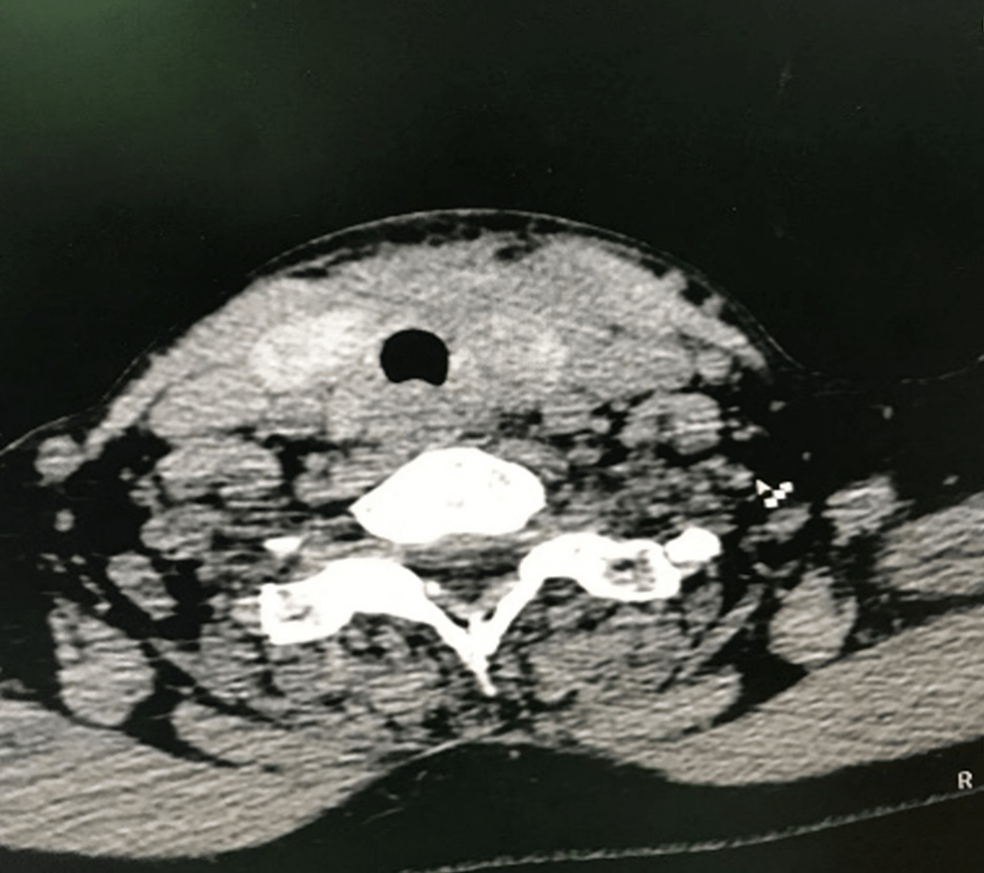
CT scan of the neck The image showed high-density lesions at the thyroid bed CT: computed tomography
